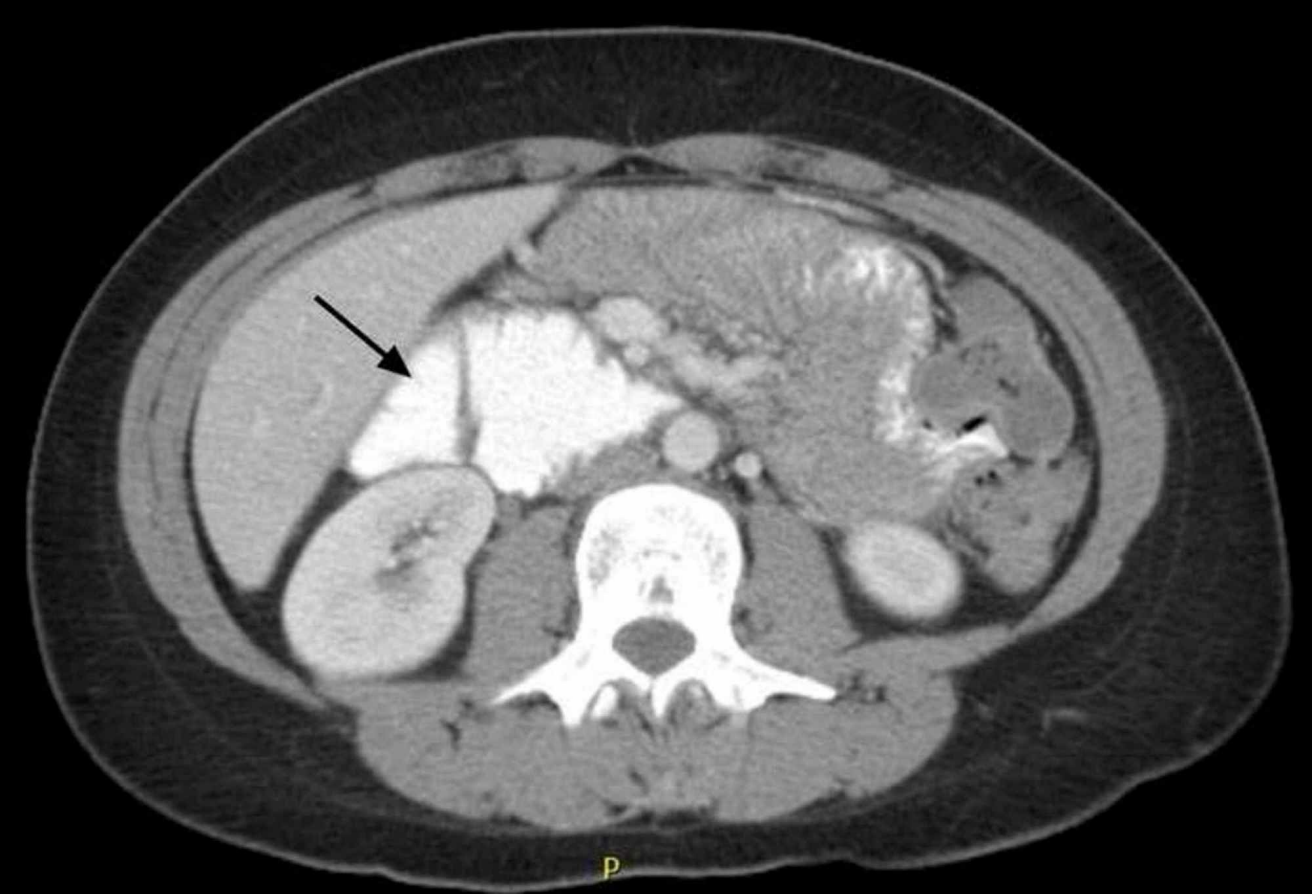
Coronal computed tomography image revealing dilated second portion of the duodenum (arrow)
